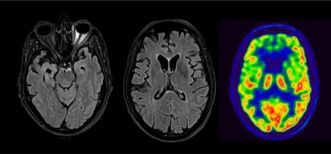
FIGURE 1 Structural MRI and FDG‐PET reveal right‐predominant frontotemporal atrophy and corresponding hypometabolism, with additional impairment of the caudate nucleus.
